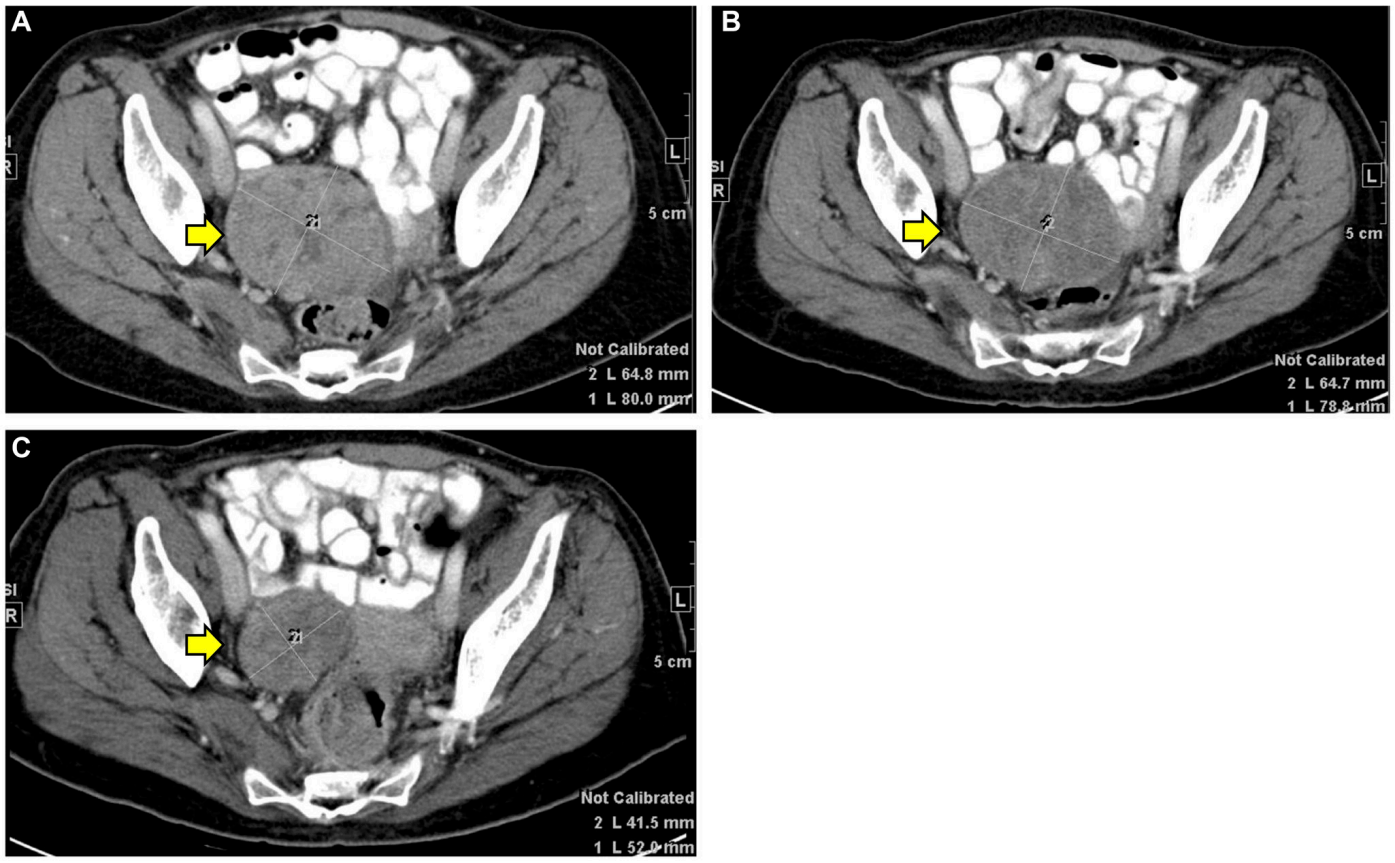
CT imaging findings of patient #9. (A) before starting immunotherapy, (B) at the start of immunotherapy, and (C) at maximal response to immunotherapy.
